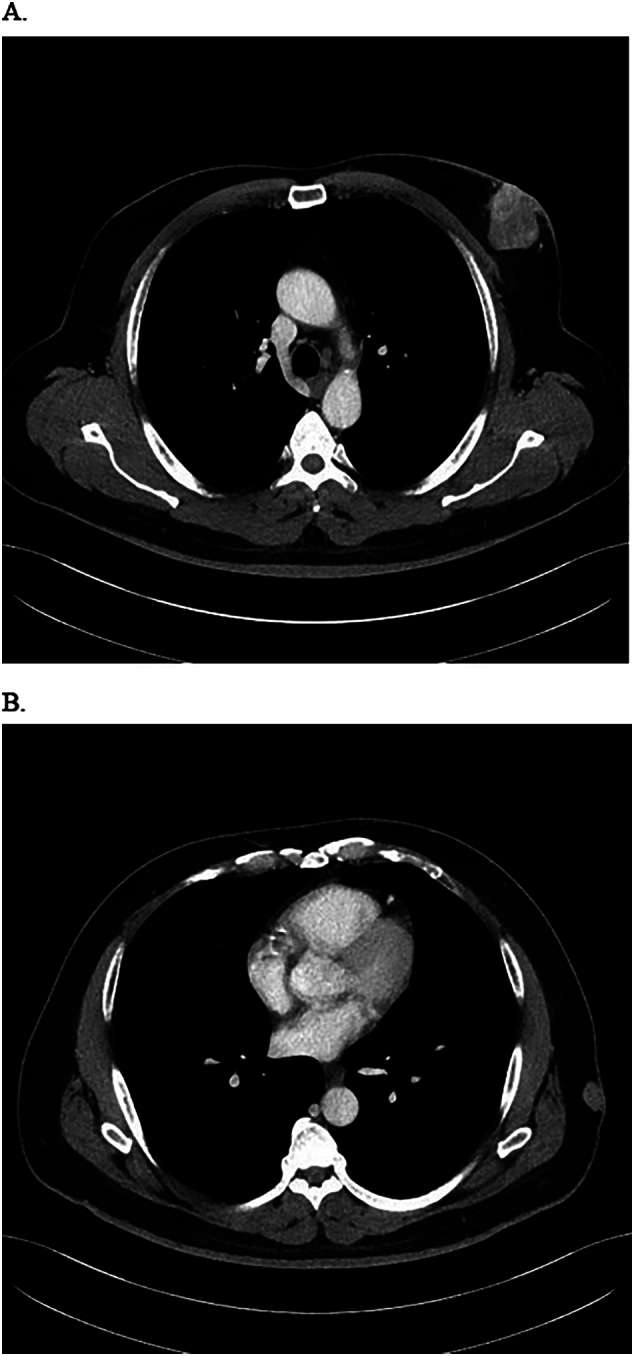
A. Chest CT scan showing left breast hyperdense lesion. B. Chest CT scan showing left lateral chest wall hyperdense lesion.
